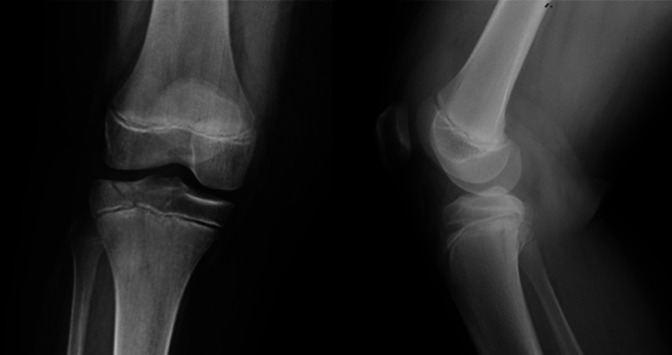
Radiographs of the knee showed a Salter-Harris 4 fracture of the proximal tibia with 4-mm displacement of the epiphysis in the coronal plane and a minimally displaced posterior metaphyseal fracture in the sagittal plane.
